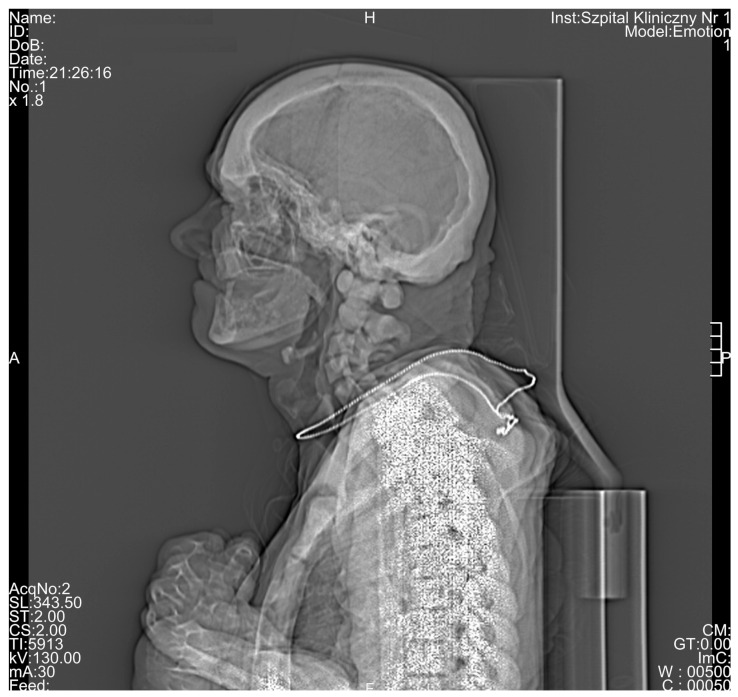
Scout view topogram obtained using computed tomography showing visible general osteosclerosis, especially within the skull base, the skull vault, and sternum.
